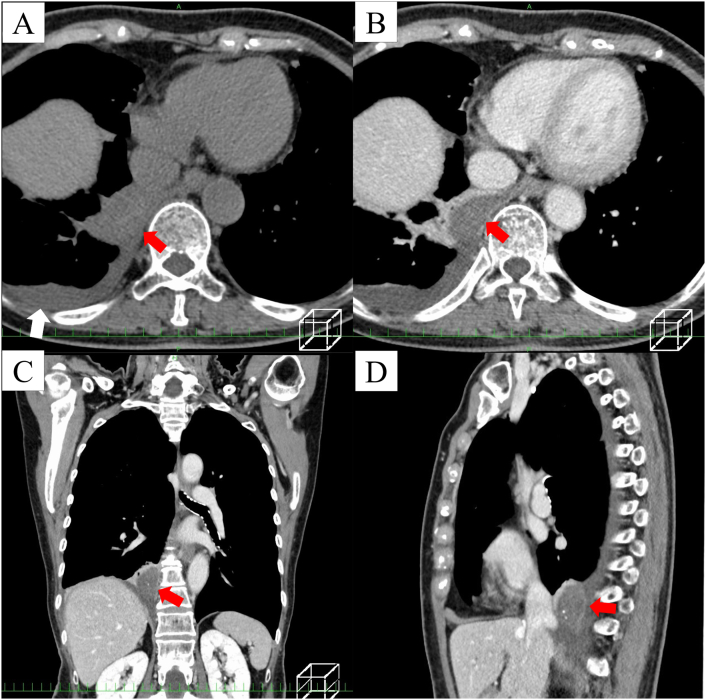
Chest computed tomography (CT) findings. (A) A paraspinal mass is visible in the right posterior mediastinum (red arrow), along with pleural effusion (white arrow). (B) Contrast-enhanced scan showing a well-defined, non-enhancing soft tissue mass in the inferior medial right pleural space, as well as mild left pleural effusion. (C) Chest coronal CT image showing a well-defined soft tissue mass (red arrow) between the diaphragm and the spine in the inferior medial right pleural space without a clear feeding vessel. (D) Chest sagittal CT image showing a mass with slight marginal enhancement (red arrow). (For interpretation of the references to colour in this figure legend, the reader is referred to the web version of this article.)
